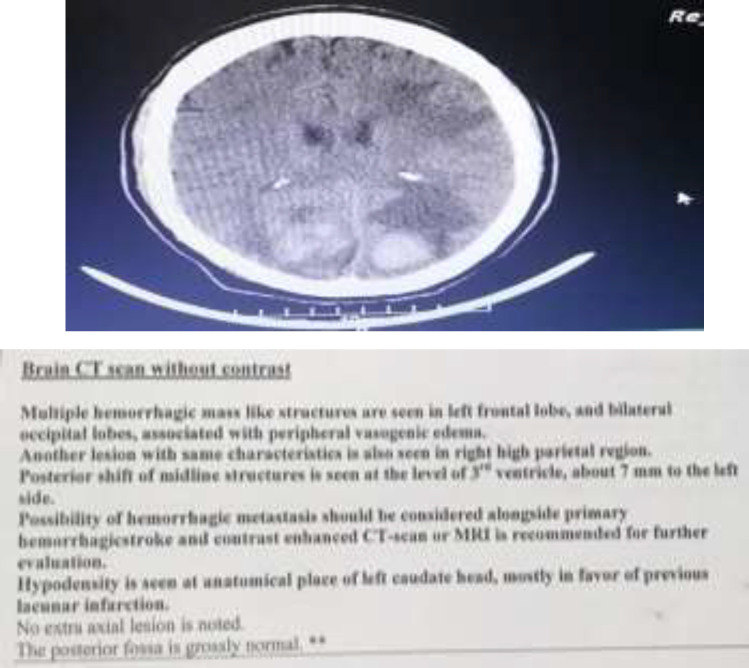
Brain CT of the patient with Myxosarcoma
